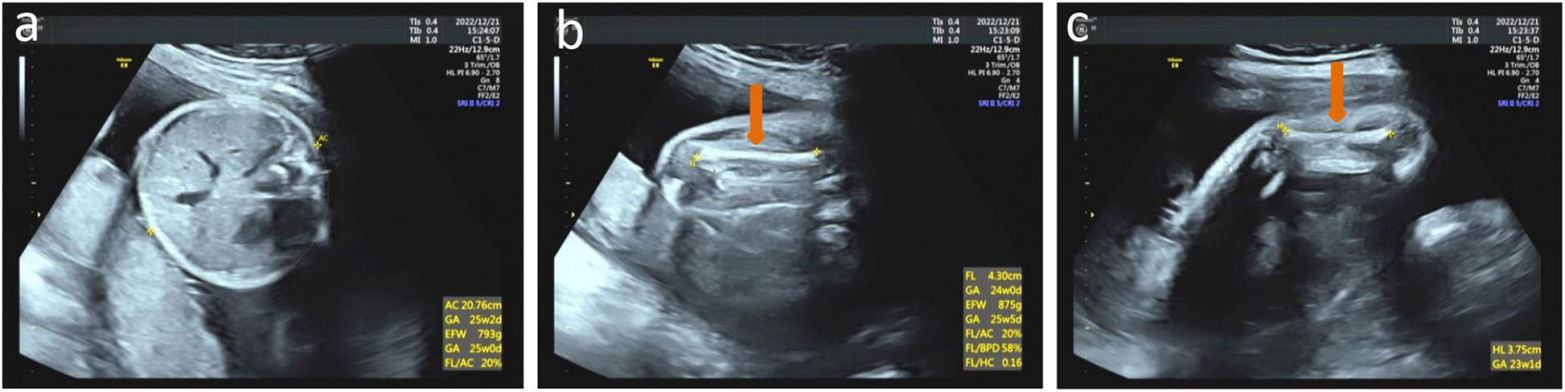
Ultrasound features of the fetus in patient 4. Narrow abdominal circumference is revealed (a). Short femurs (b) and humeri (c) are indicated with red arrows
